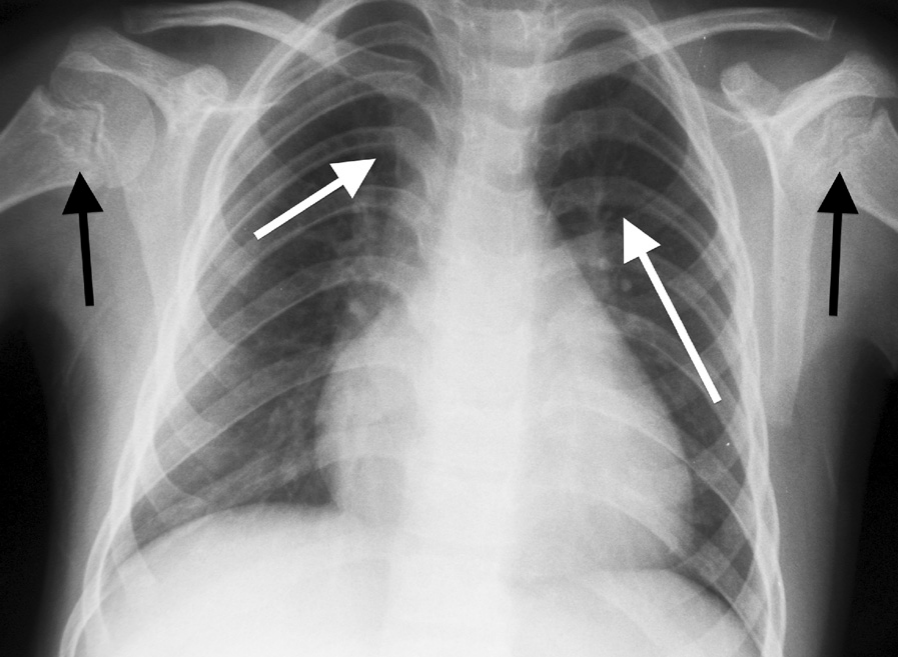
Chest radiograph of UPN1 at referral for BMT aged 11 years. There is mild metaphyseal sclerosis and irregularity of the proximal humeri (black arrows). The posterior ribs are bowed (white arrows) and there is the impression of cardiomegaly due to the slightly narrowed thorax.
